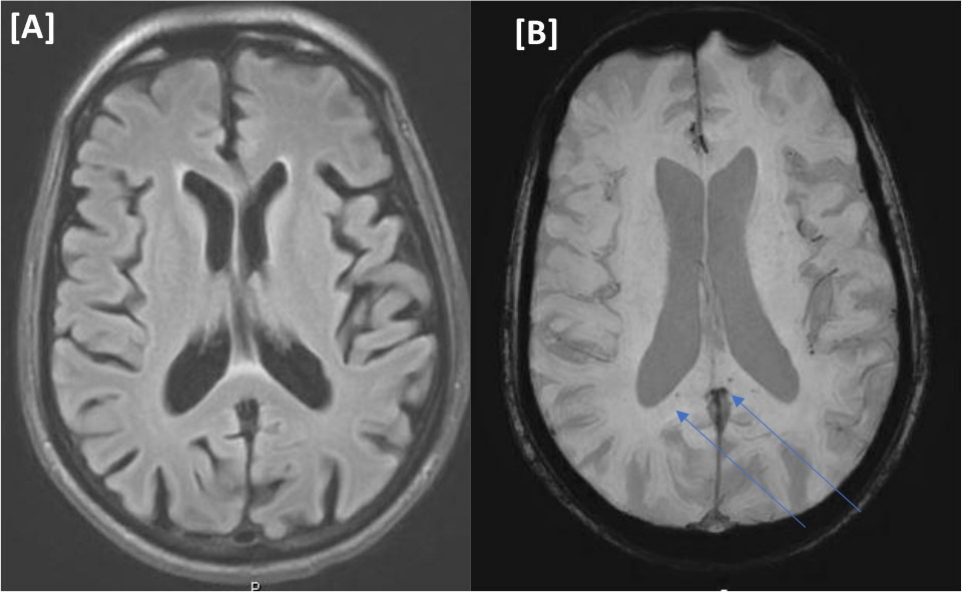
(Case IV) [A and B images] Magnetic resonance imaging of the head. No focal parenchymal abnormal signal intensity on FLAIR (A). Few punctate foci of microbleeds at the splenium of the corpus callosum on susceptibility-weighted images in panel (B).
